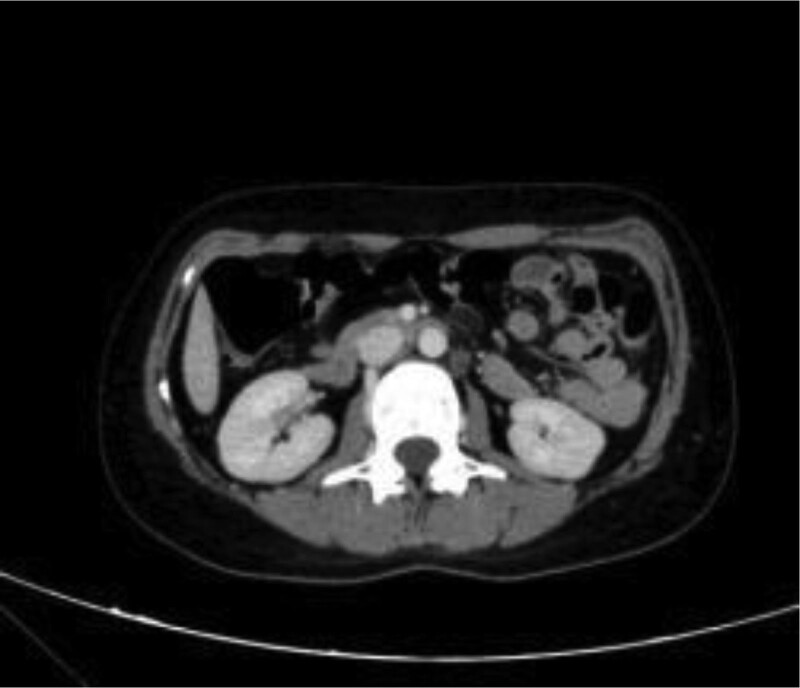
Total abdominal CT plain scan + triple-stage enhancement.
